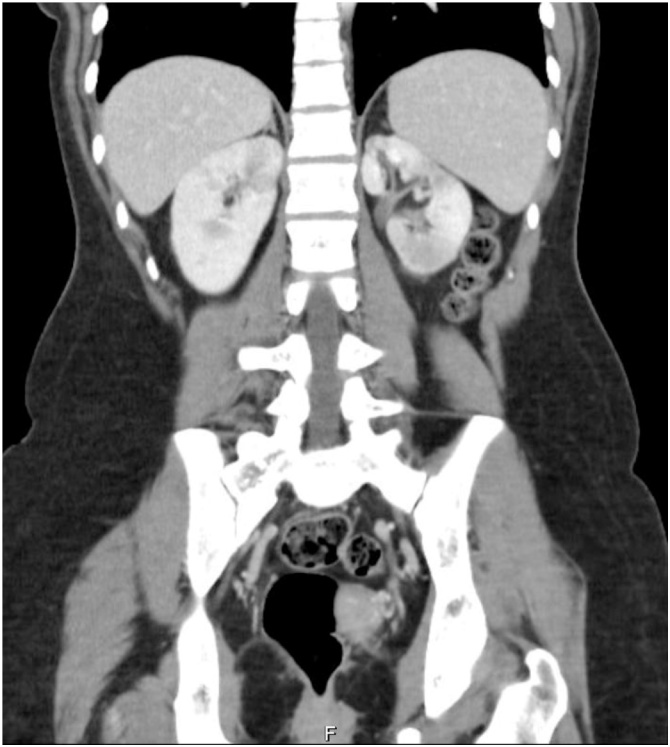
CT scan, sagital view, showing a heterogeneous uptake of intravenous contrast in left kidney in favor of pyelonephritis.
